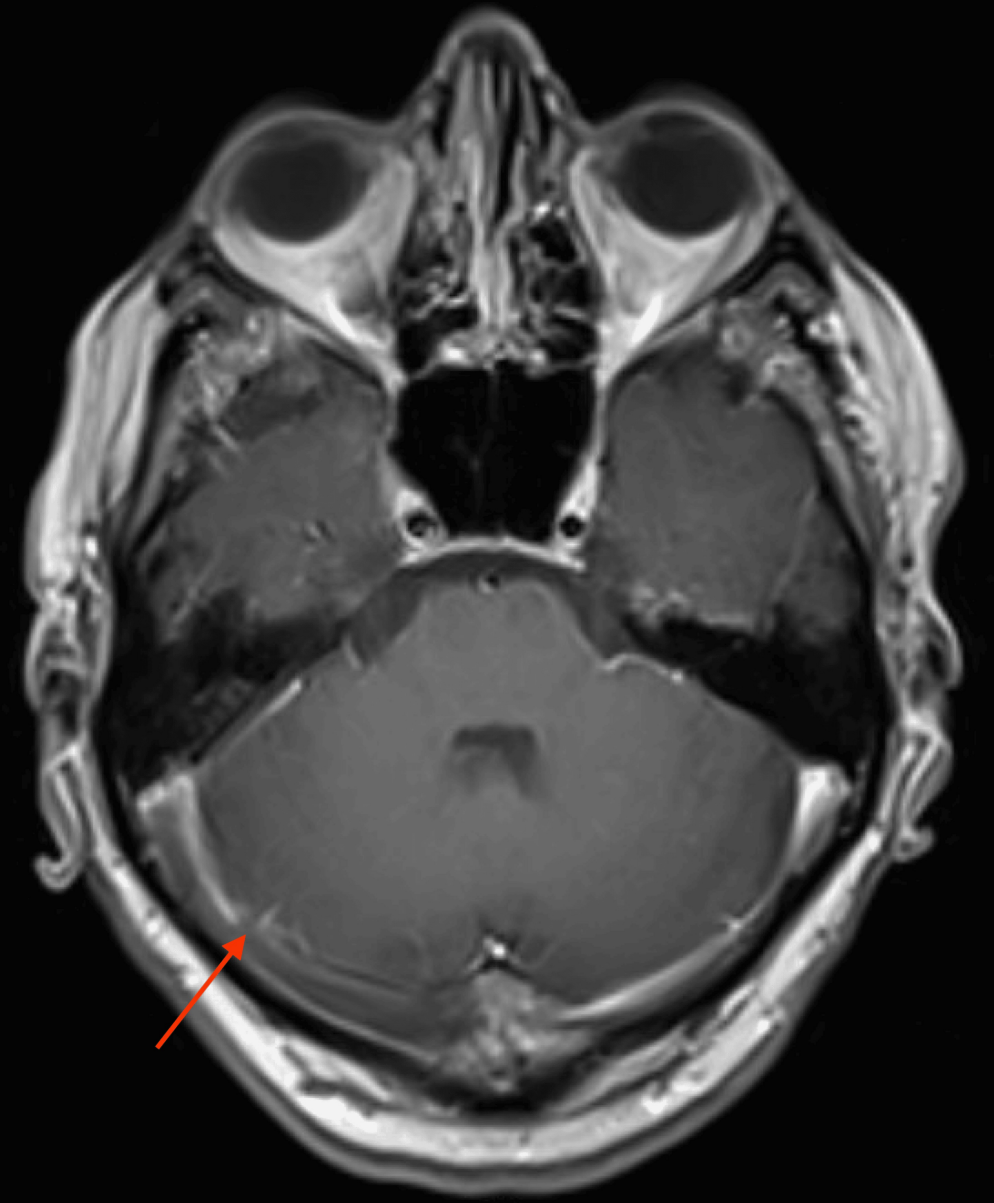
MRI of the brain with contrast, axial view, showing near-complete thrombosis of the superior sagittal sinus with extension into the right transverse sinus (arrow)
